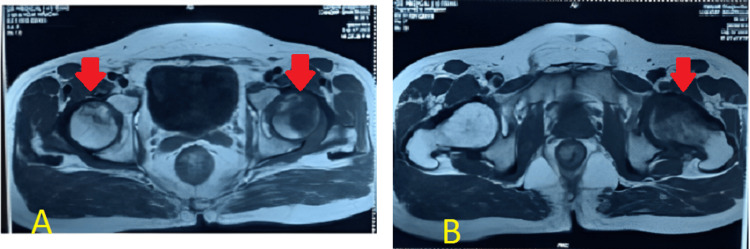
Axial section (A) and (B) of MRI, both hips showing more destruction on the left side as compared to the right The axial slices of images A and B show avascular necrosis, or the loss of vascularity, on both sides, with the left side showing more of it.
